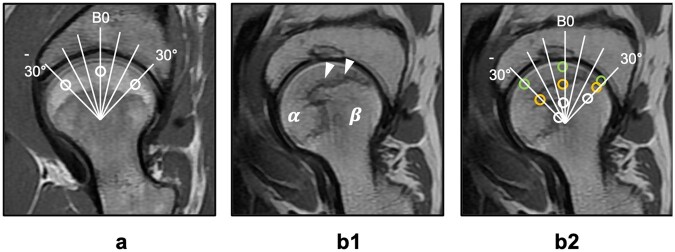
Division of the femoral head in T1WI images and ROI selection. The ROIs were used to divide the IDEAL-IQ and IVIM-DWI images of the femoral head into radial sections. (a) For the normal hips and contralateral asymptomatic hips, the center was confirmed using a concentric circle in the femoral head and a center line parallel to B0 that passed through the center of the femoral head (white circle: normal area selection). (b1) In SIONFH, the femoral head is divided into three parts, i.e. the normal area (β), penumbra area (white arrow, presenting as a low signal band) and necrotic area (α, presenting as a high signal). (b2) ROIs were allowed to be adjusted no more than 5° if the necrotic or penumbra area was not big enough (green circle: necrotic area selection; orange circle: penumbra area selection; white circle: normal area selection).
